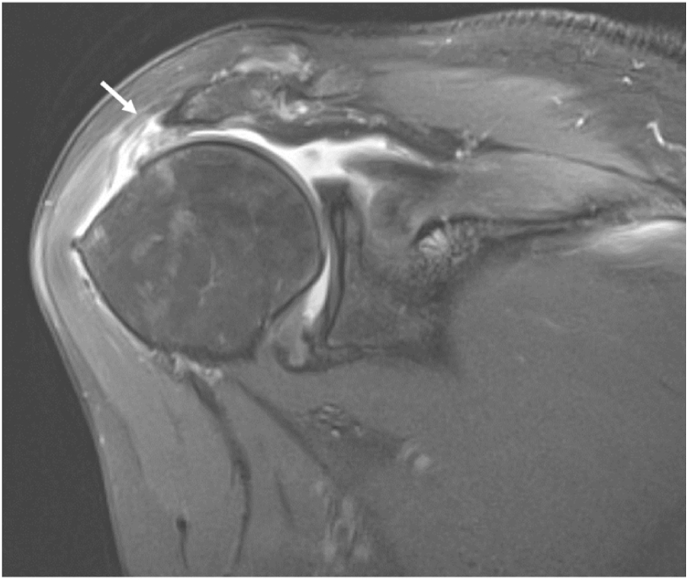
Coronal proton density fat-saturation MR image exhibits partial undersurface tear of the middle portion of the deltoid muscle (white arrow) at its acromial insertion, in association with a massive retracted full-thickness tear of the supraspinatus tendon. Note the proximal migration of the humeral head and the reduced acromio-humeral interval.
